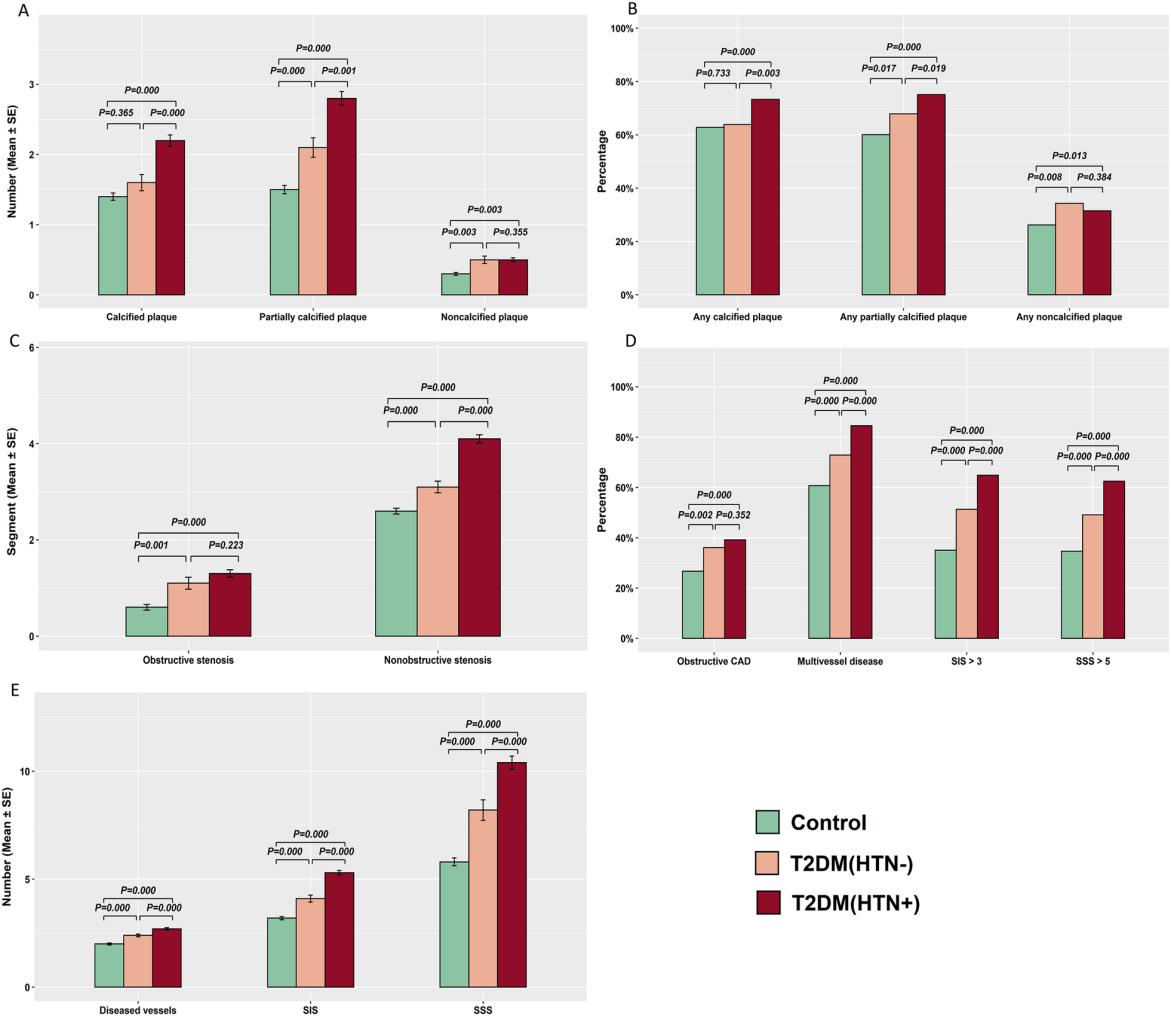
Coronary plaque burden, stenosis and extent of coronary artery plaques detected by coronary computed tomography angiography. The mean value (A) and proportion of any presence (B) of different plaque types; the mean value of obstructive and nonobstructive coronary artery disease (CAD) (C); the proportion of any presence of obstructive CAD, multivessel disease, segment involvement score (SIS) > 3 and segment stenosis score (SSS)  > 5 (D); the mean value of diseased vessels, SIS and SSS (E)
